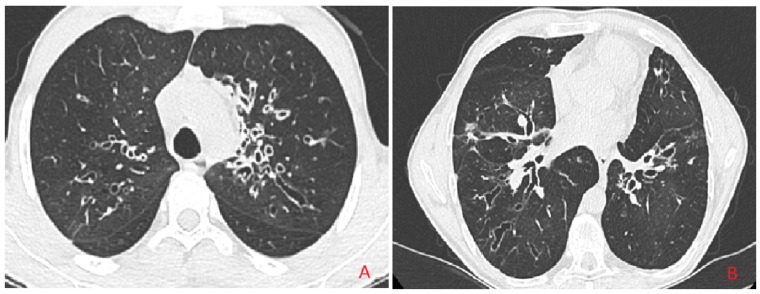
(A) Axial chest CT of a patient with cystic fibrosis demonstrating bilateral bronchiectatic changes, predominantly involving the left lung, with associated peribronchial wall thickening and mucus plugging. (B) Axial chest CT of a patient with more advanced disease, showing extensive bilateral cylindrical and saccular bronchiectasis, marked peribronchial thickening, and mucus plugging.
